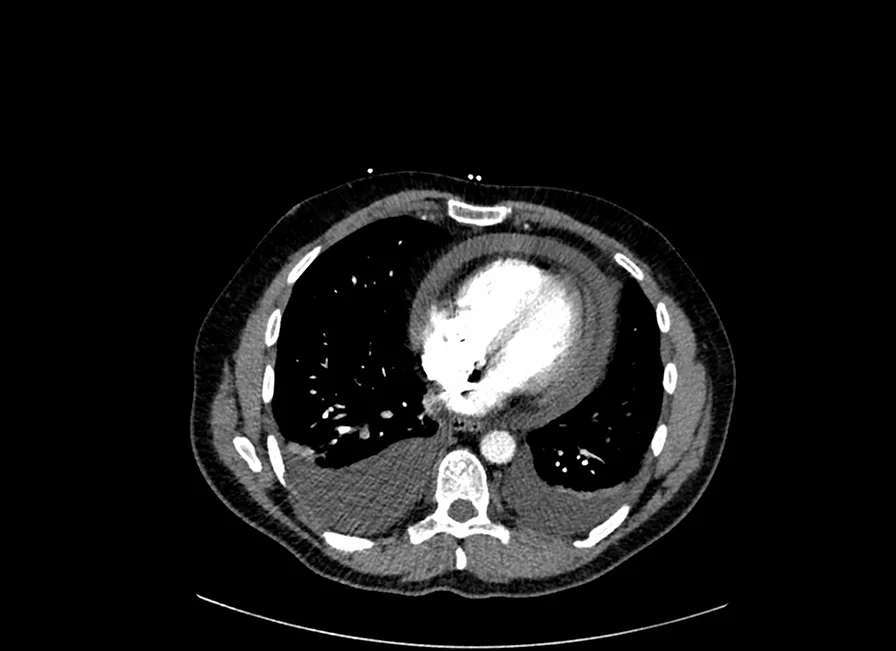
CT chest showing large bilateral pleural effusions as well as pericardial effusion and thickened pericardium
